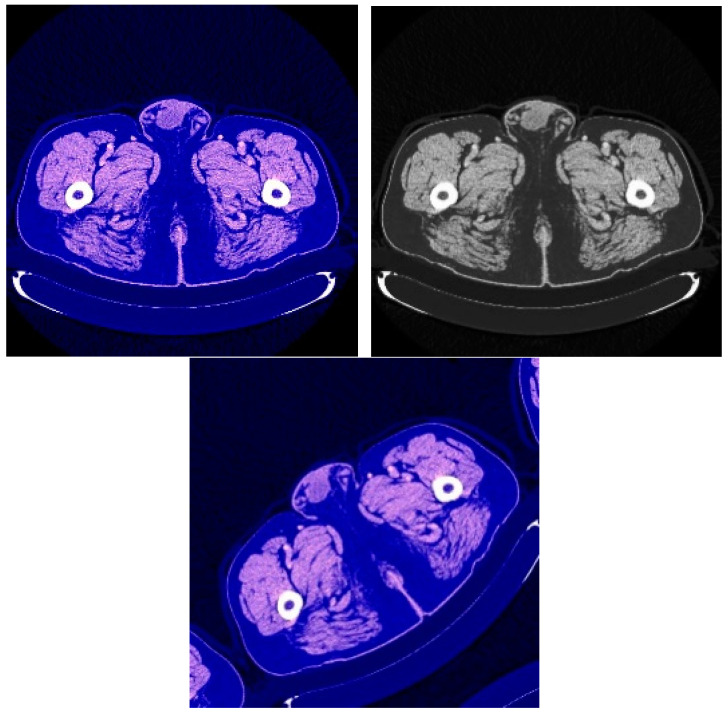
Examples of the input CT images after applying augmentation.
